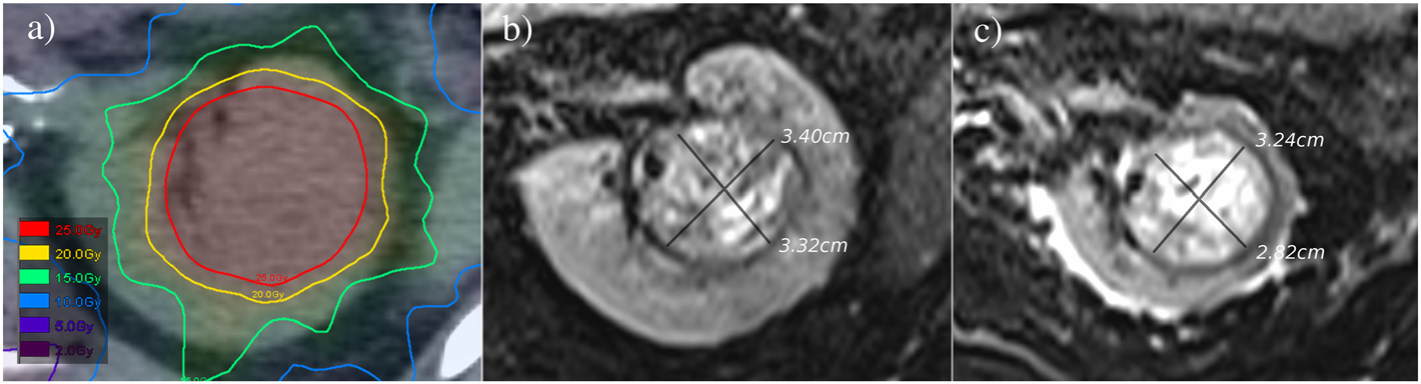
Axial images of renal carcinoma a) on baseline planning CT, b) pre-therapy T2-weighted MRI, & c) T2 MRI at 18 months post-SABR.
